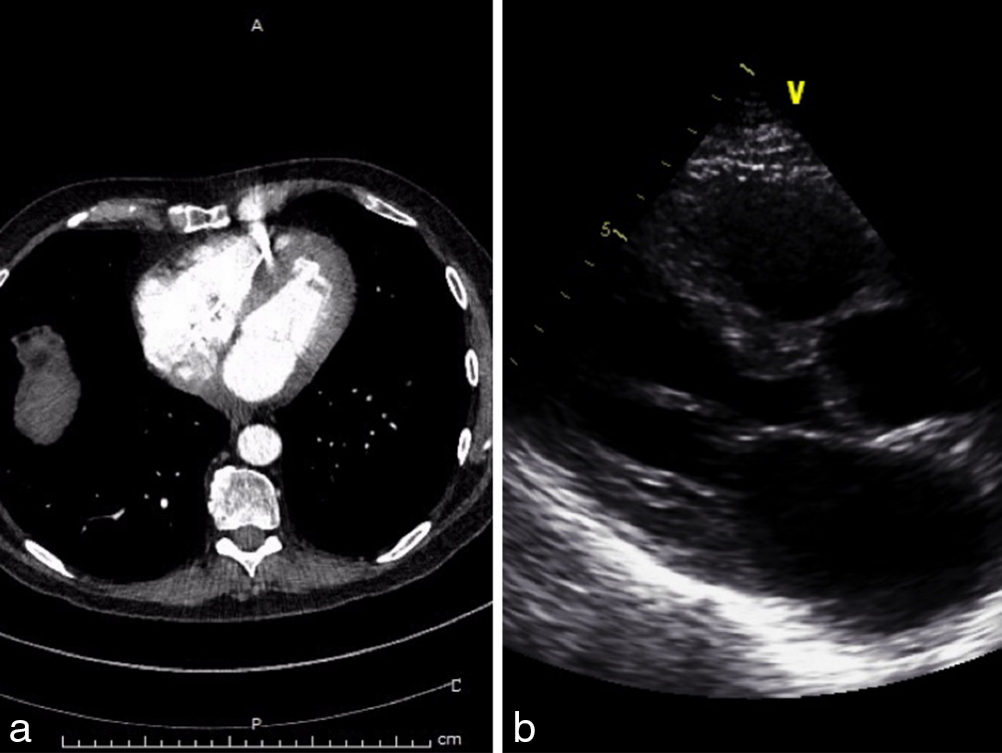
(a) CT of the thorax showing significant improvement in the appearance of the lymphoma following chemotherapy. (b) Transthoracic echocardiogram (parasternal long axis view) showing significant improvement in the appearance of cardiac chambers post chemotherapy.
